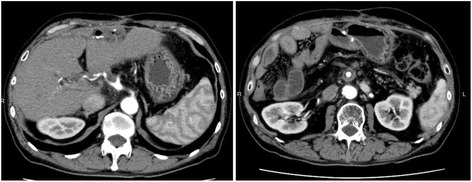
The abdominal computed tomography scan in April 2016, about 20 months after the radiation therapy, shows no relapse of the recurrent lesions
